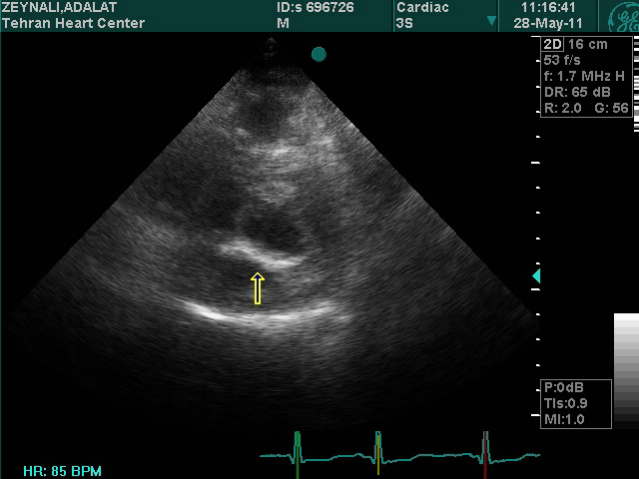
Parasternal short-axis view of mitral annulus calcification (MAC) by transthoracic echocardiography.
